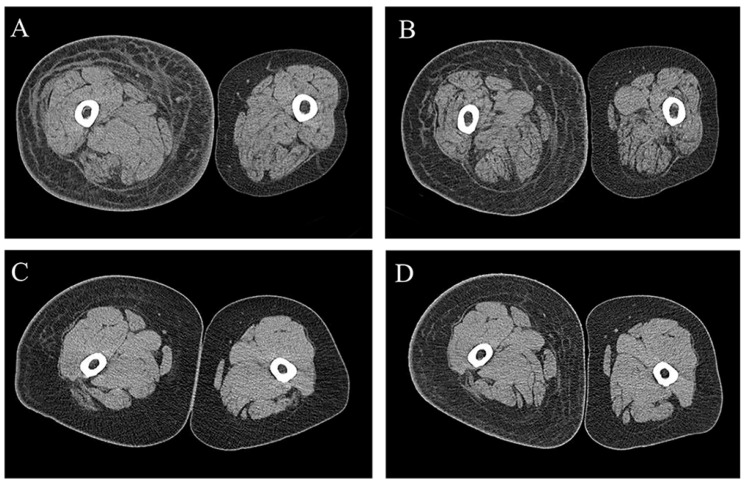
Bidirectional change of subcutaneous fibrosis in computed tomography (CT) scan. Fibrosis was improved in follow-up (B) than initial (A). Fibrosis was aggravated in follow-up (D) than initial (C).
